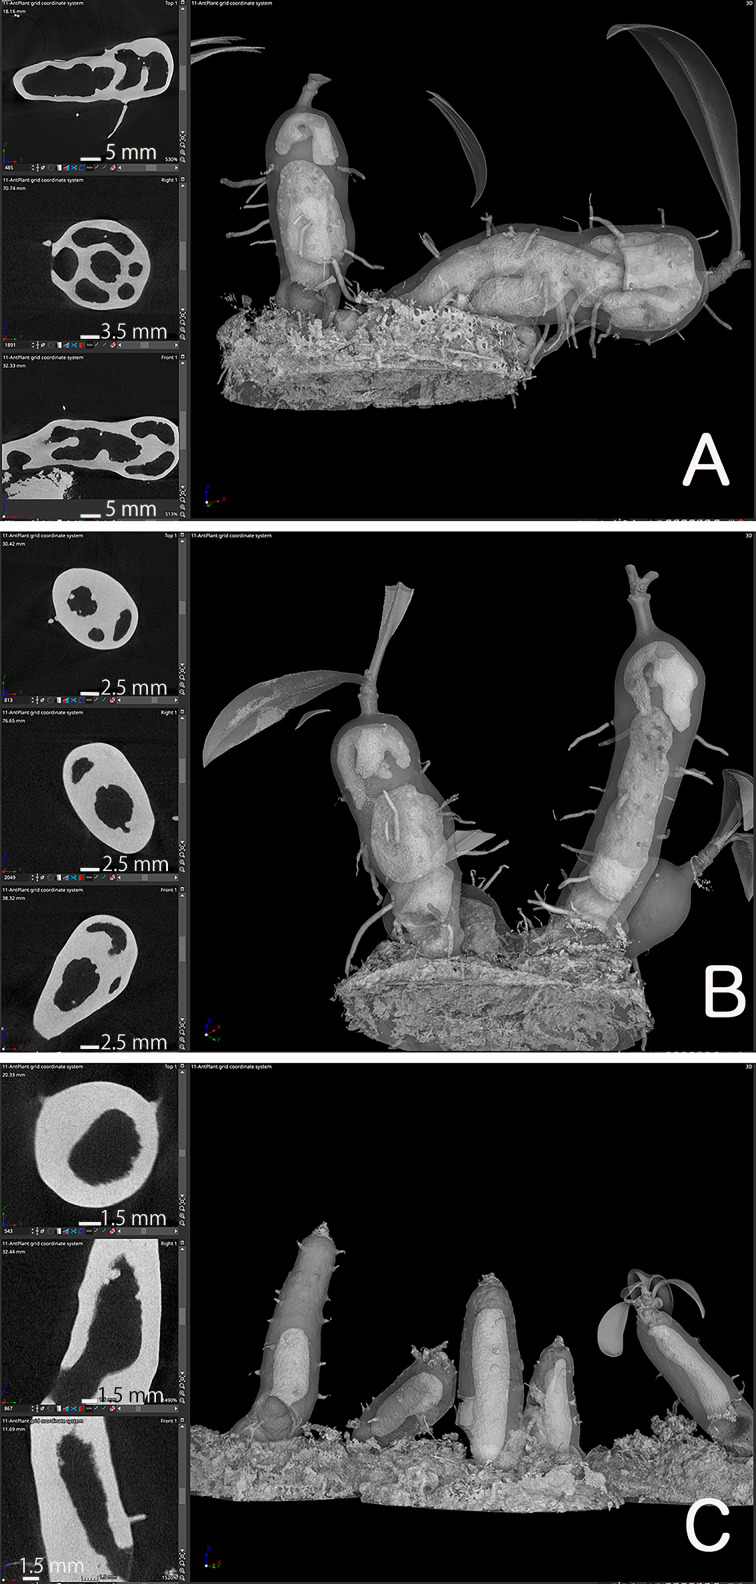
Cavity shape variations. Micro computed tomography images of (A, B)
